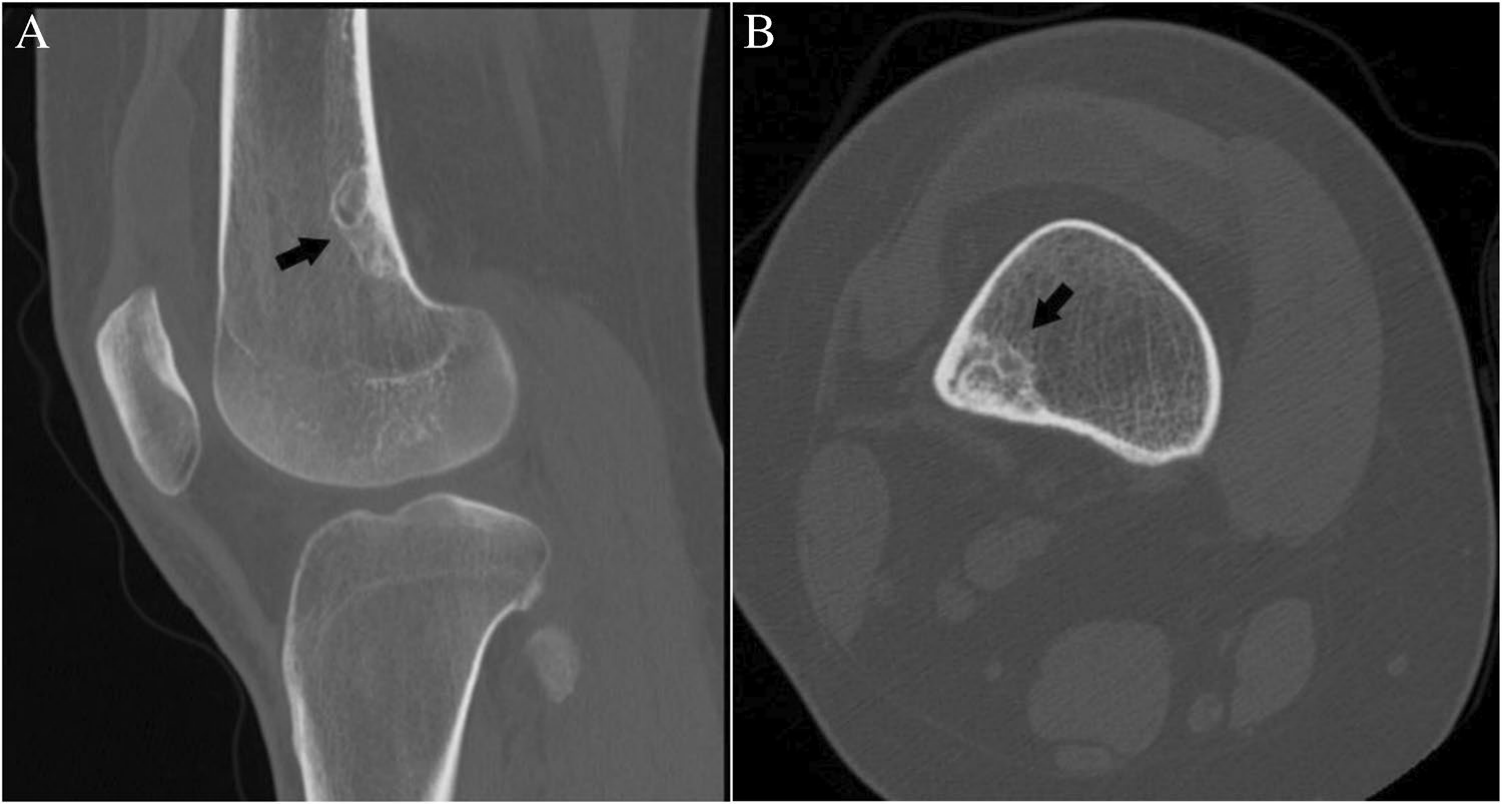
Case 6. Non-ossifying fibroma. 21-year-old man with medial knee pain. A Sagittal and B axial CT images show a mixed density cortically based lesion (arrows) with in the distal femur without concerning features
